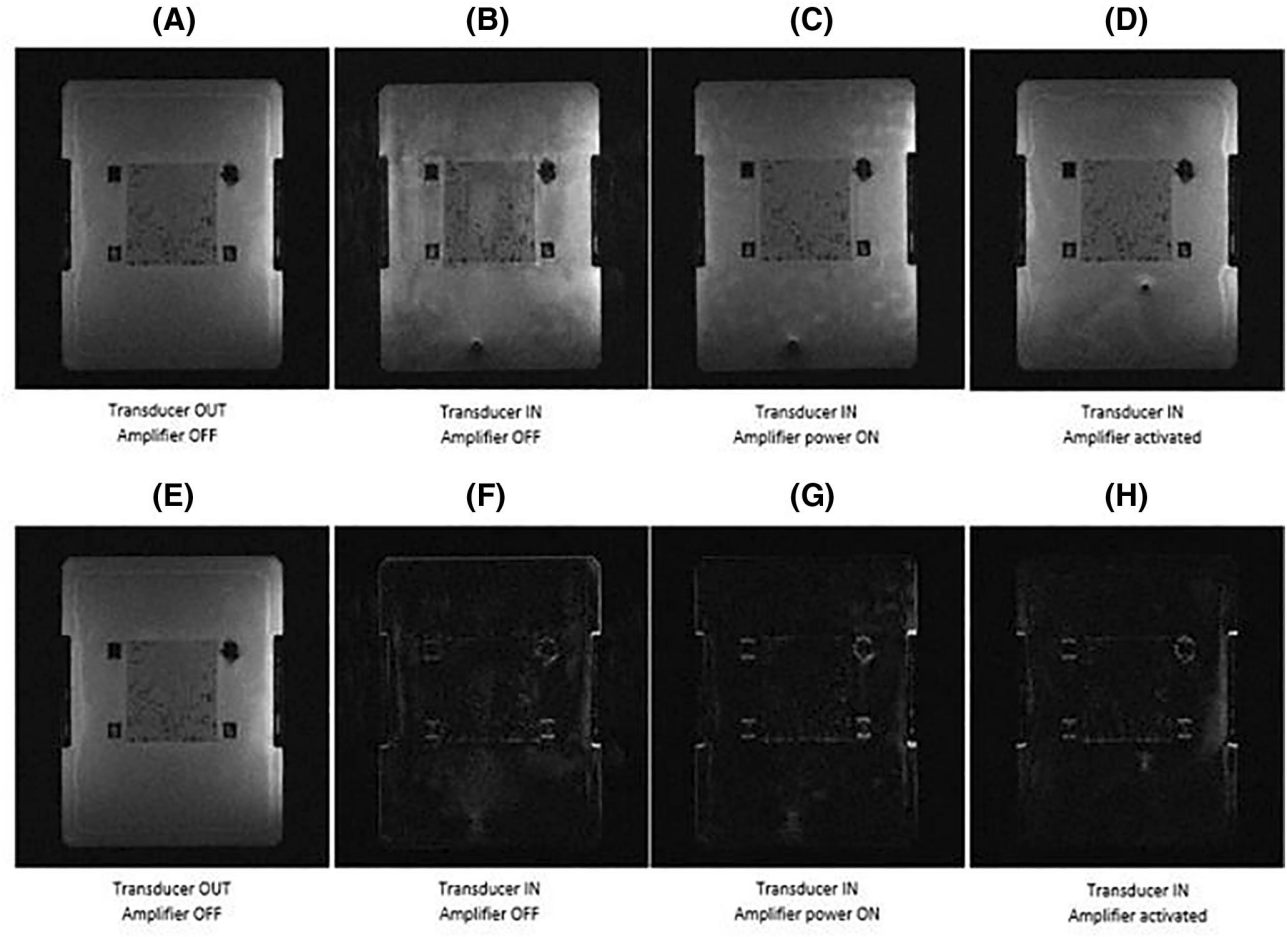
MR images (A–D) obtained using FSPGR for different activation conditions of the transducer and their corresponding differences (E–H) from the baseline image. FRFSE, Fast Relaxation Fast Spin Echo; MR, magnetic resonance
